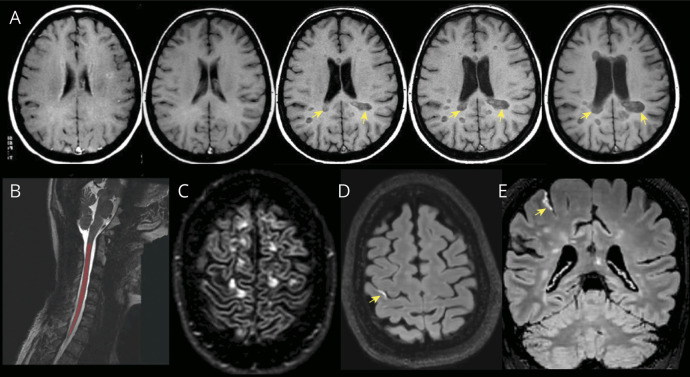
Examples of Brain Atrophy, Slowly Expanding Lesions, Spinal Cord Segmentation, Cortical Lesions, and Leptomeningeal Enhancement (A) Axial T1-weighted images acquired between 1995 (first image on the left) and 2005 (last image on the right) showing progressing development of brain atrophy (increase size of the ventricles and widening cortical sulci) and slowly expanding lesions (yellow arrows). (B) Sagittal T2-weighted image showing one of the steps required for computation of spinal cord longitudinal atrophy, which involves segmentation of the spinal cord (area in red) which is separated from the cerebro-spinal fluid. (C) Axial DIR image showing a couple of intracortical lesions (yellow arrows). (D) Axial and (E) coronal contrast-enhanced 3D T2-FLAIR images showing a high-signal linear hyperintensity (yellow arrow) in the right central sulcus, indicating leptomeningeal enhancement. DIR = double inversion recovery; FLAIR = fluid-attenuated inversion recovery.
